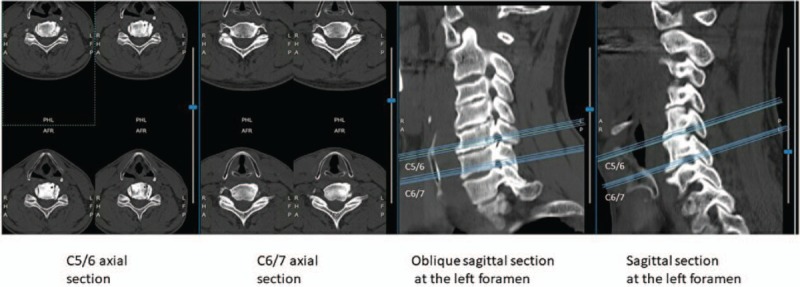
Oblique sagittal computed tomography imaging confirms the presence of left C5/6/7 foraminal stenoses. Axial slices are of the C5/6 and C6/7 levels. Oblique sagittal and sagittal slices are of the left foramen.
